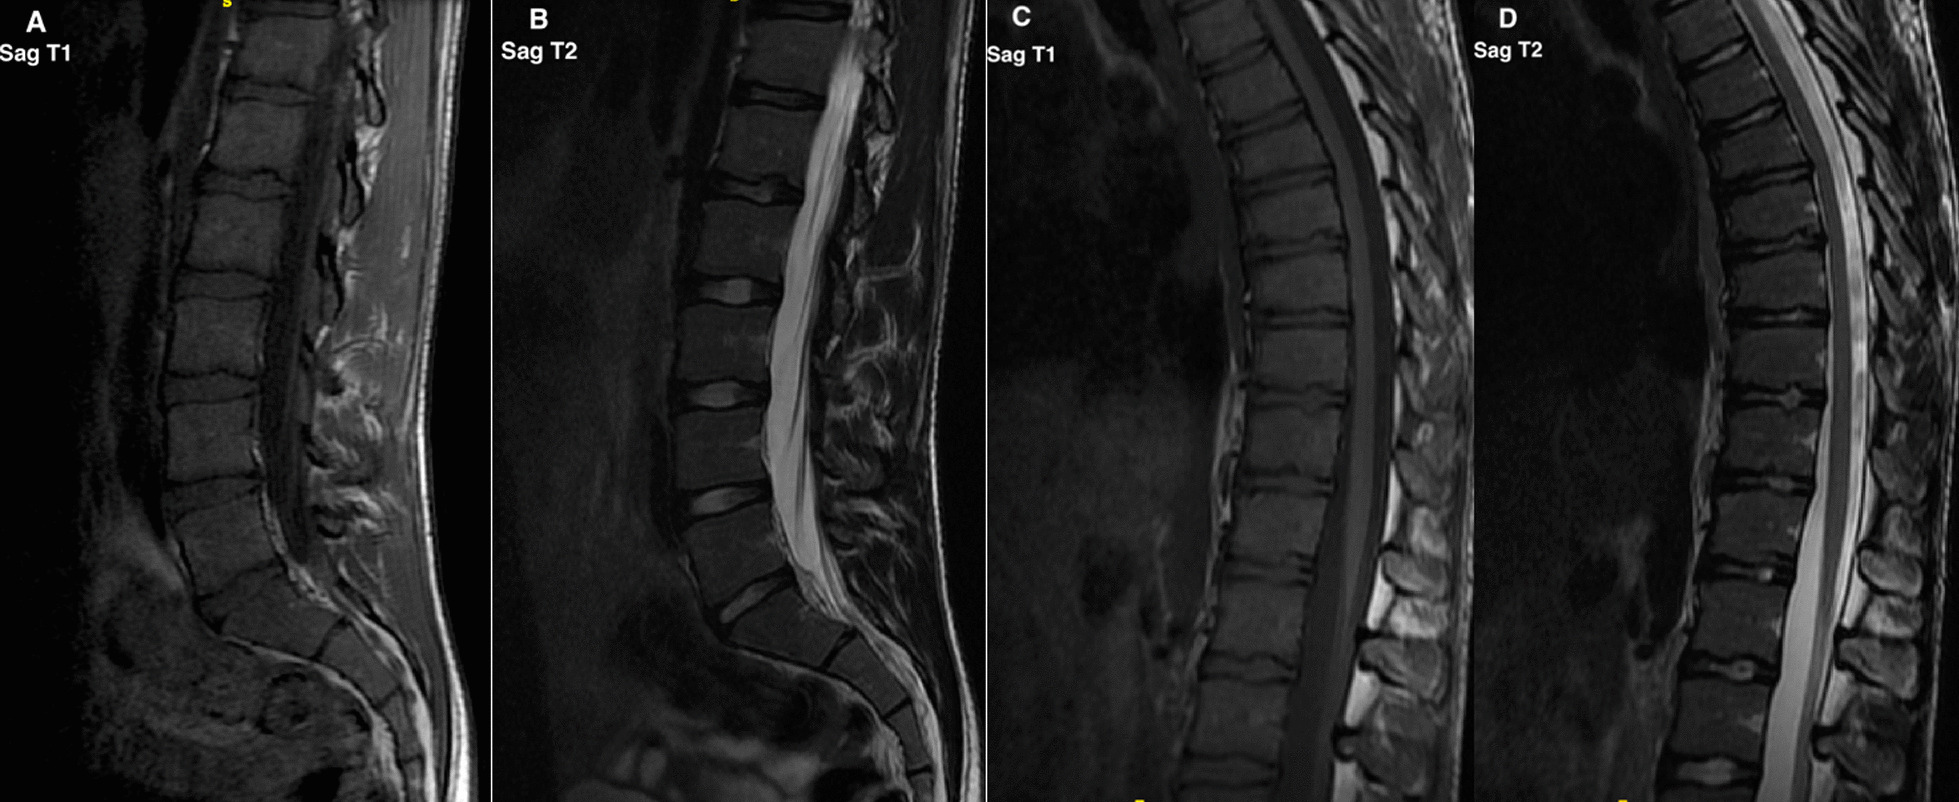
MRI of a male climber in the 20s, with the highest accumulated number of spinal changes of the climbing participants. The lumbar imaging (A + B) displays endplate changes of the Th12-L1 segment. The thoracic imaging (C + D) displays multiple spinal segments with low grade endplate changes and reduced disc signal intensity. This individual constituted an outlier in the sample, with endplate irregularities commonly seen in Scheuermann´s disease. The participant did not however fulfil the criteria for Scheuermann´s disease
